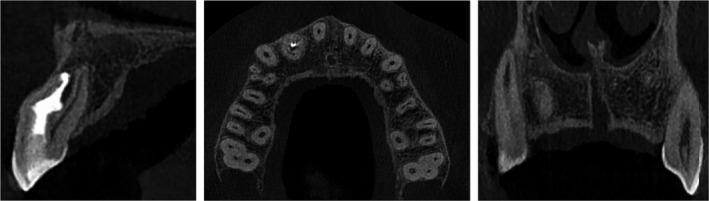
Cone‐beam computed tomographic (CBCT) imaging in 24‐month follow‐up shows total reparation of bone structures
